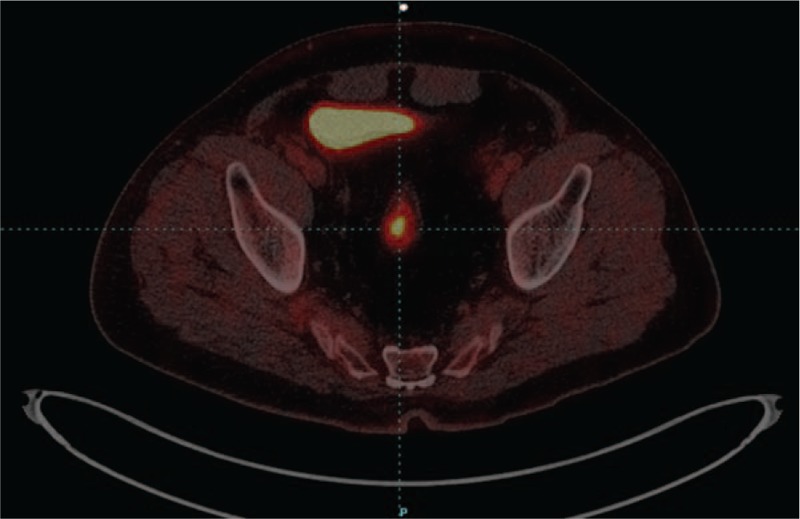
Axial view of FDG-PET/CT: hypermetabolic activity in upper rectum with average SUV of 5.0 and SUVmax of 10.0, 1.6 cm × 2.8 cm × 1.8 cm sizes.
